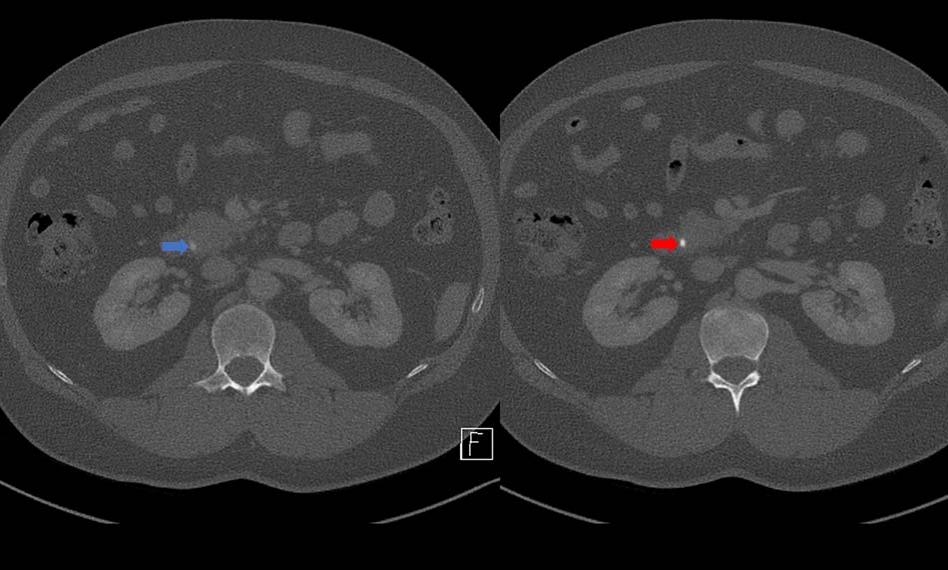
CT demonstrating an obstructing calculus (right image) present within the CBD distinct from the limy bile (left image) (WW 2249, WL 450).
